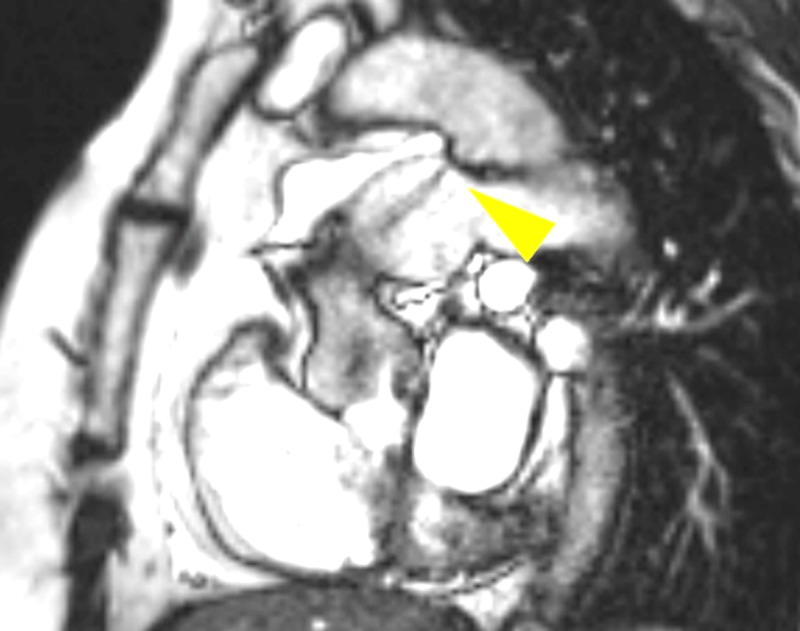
Cardiac magnetic resonance imaging showing patent ductus arteriosus with left-to-right shunting. Yellow triangle indicates flow jet from the aorta to the pulmonary artery.
